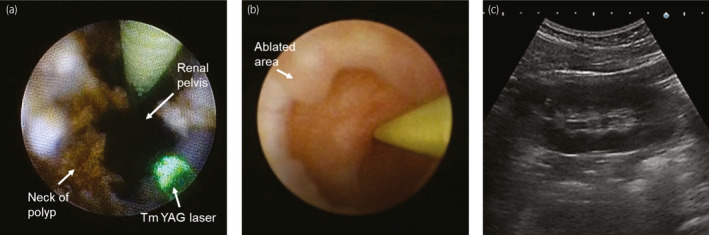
(a) Endoscopic imaging of resection of the UFEP with Tm:YAG laser. (b) Second‐look URS showing no recurrent UFEPs or ureteral stricture 8 weeks after the first URS. (c) Ultrasonography representing no hydronephrosis at 1‐year follow‐up. Tm:YAG, thulium:yttrium aluminum garnet; UFEP, ureteral fibroepithelial polyp; URS, ureteroscopy.
